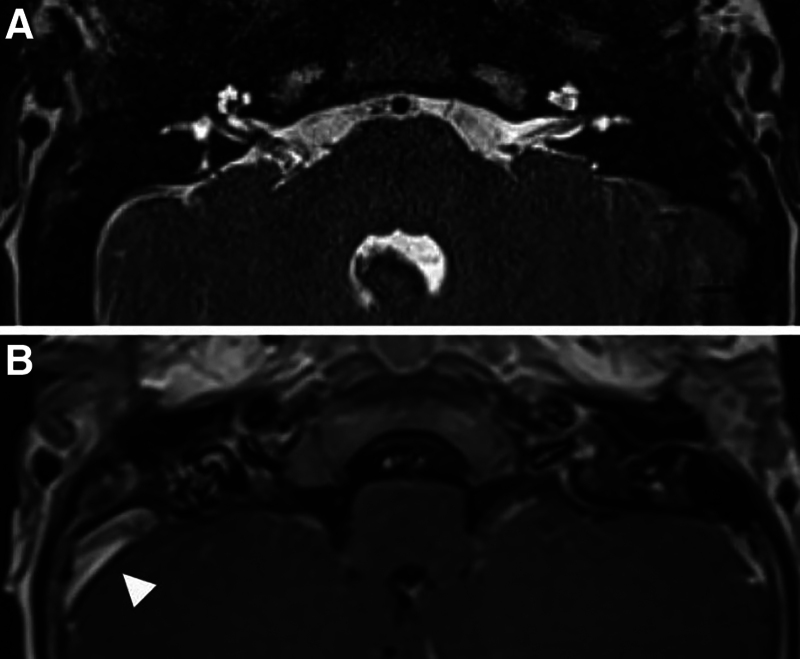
MRI of the IAC. *A*, constructive interference in steady state axial image demonstrating normal-appearing cochlea and inner ears bilaterally. *B*, T1 axial post-contrast showing anterior and dominant sigmoid sinus on the right (arrow). IAC indicates internal auditory canal.
